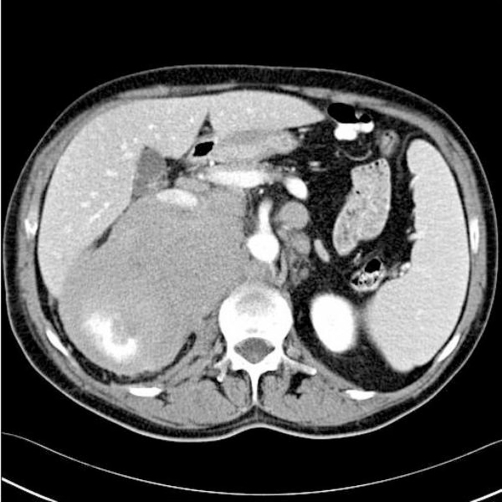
Abdominal CT (contrast enhanced) scan image showing hypodense renal mass involving the right kidney with perinephric extension.
